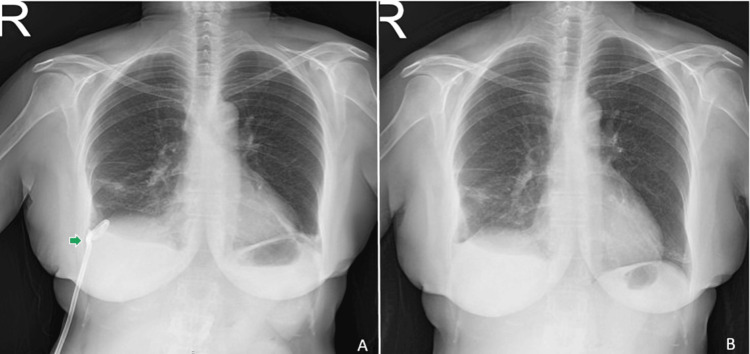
Patient's follow-up chest X-rays A: Control chest X-ray after removal of the port catheter. The pigtail catheter is highlighted by the green arrow. B: Control chest X-ray upon discharge after removal of the pigtail drainage catheter
